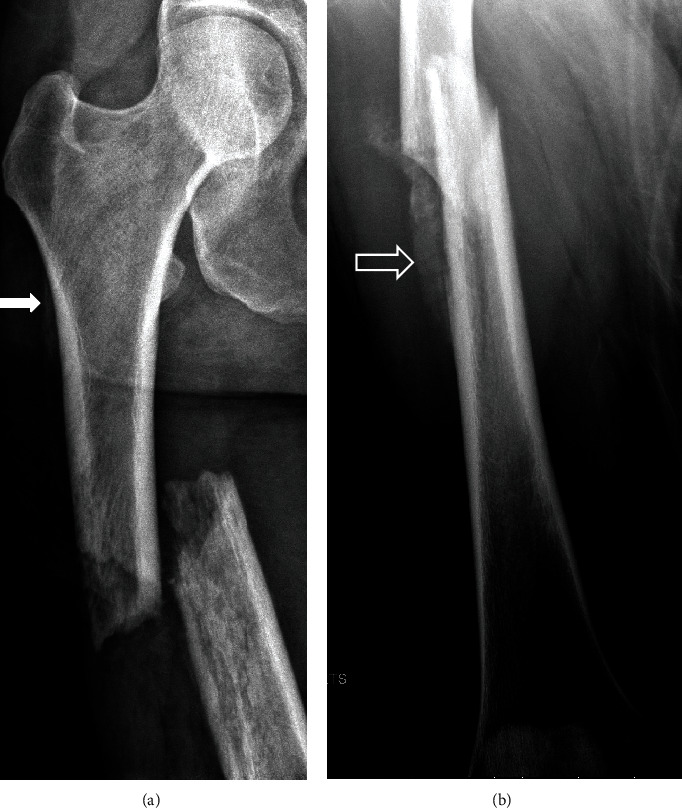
33-year-old woman with one month of vague thigh pain presented with pathologic fracture. (a) AP radiograph of the right femur shows a displaced pathologic fracture of the proximal femoral shaft. Smooth periosteal reaction (arrow) surrounds the proximal femoral shaft. Permeative bone destruction is seen at the fracture bone ends. (b) Oblique radiographic view shows additional organized, thick, and dense periosteal reaction or involucrum around the infected cortex (open arrow).
